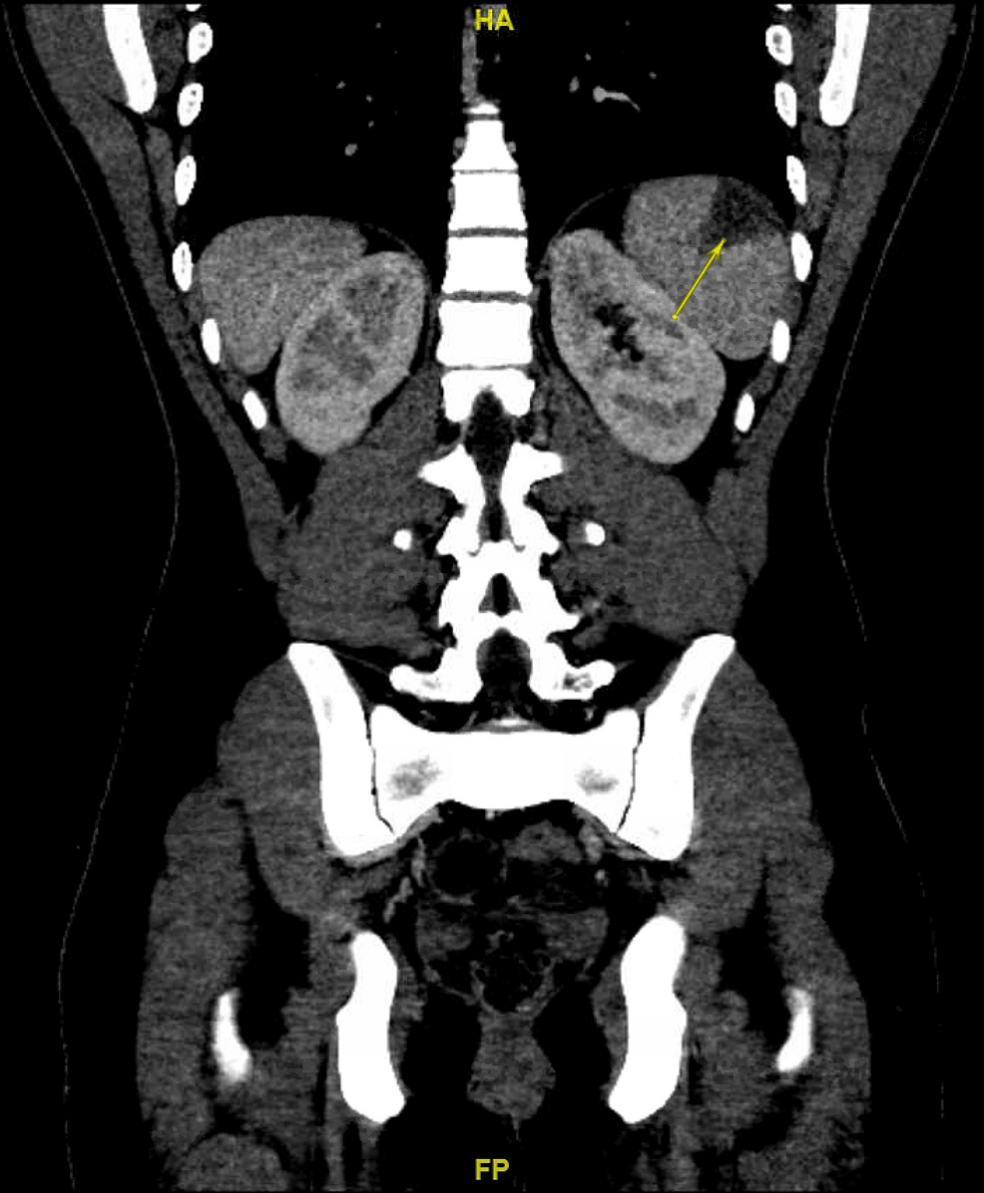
CT scan of the abdomen showing a wedge-shaped infarction in the spleen (yellow arrow).
